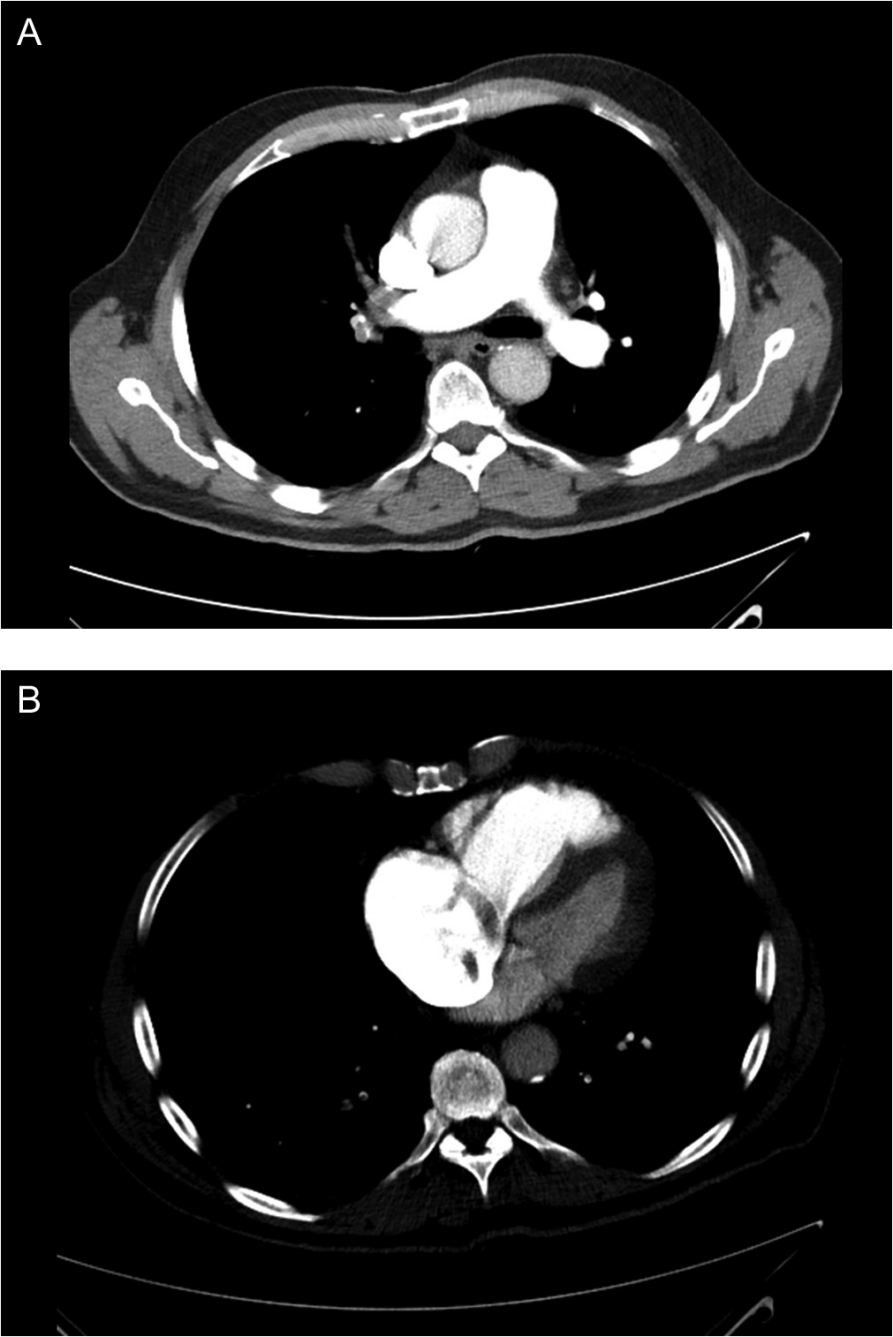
(A) CT pulmonary angiogram demonstrating emboli in the right pulmonary arteries with dilatation of the main pulmonary trunk. (B) CT pulmonary angiogram demonstrating intra-cardiac filling defects.
